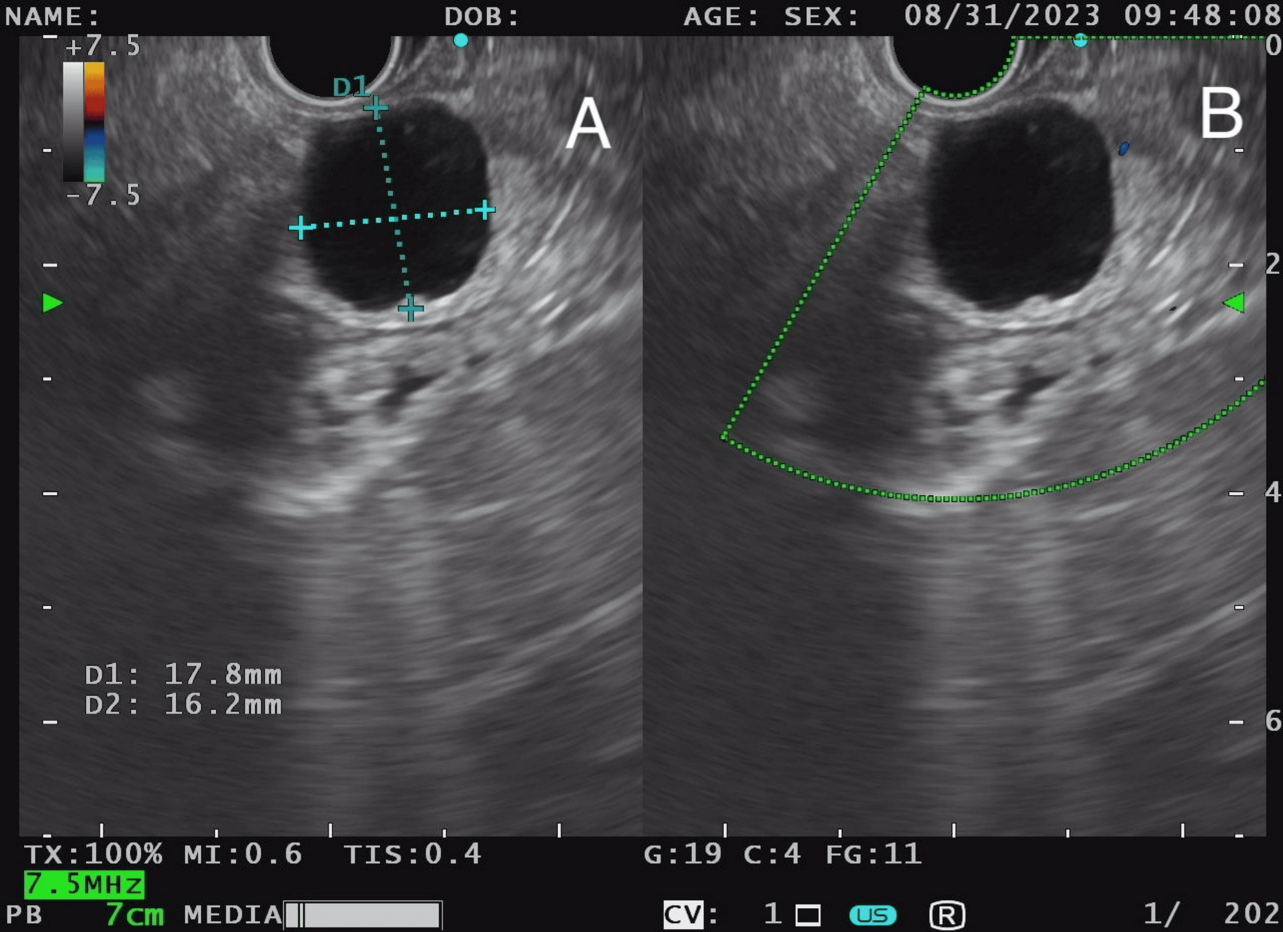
Endosonography: (A) the measurement of the pancreatic lesion and (B) the use of a 7.5 MHz transducer (A and B) Homogeneous pancreatic parenchyma with regular glandular margins. A well-defined 22 mm cyst is identified in the neck extending toward the body of the pancreas, with thin walls, no thickening or growth into the lumen, regular margins, and anechoic content
